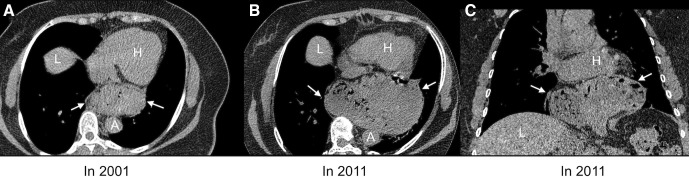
Non-contrast chest CT of a woman aged 70 years showing natural history of hiatal hernia (HH) over 10 years from Multi-Ethnic Study of Atherosclerosis (MESA) Exam 1 (2000–02) to MESA Exam 5 (2010–12). (A) Axial image of type III HH (arrows) at MESA Exam 1 (2001). (B) Increased size of type III HH (arrows) at MESA Exam 5 (2011). (C) Coronal reformation at MESA Exam 5 showing most of stomach herniated into thorax (arrows). A, aorta; H, heart; L, liver; S, stomach.
